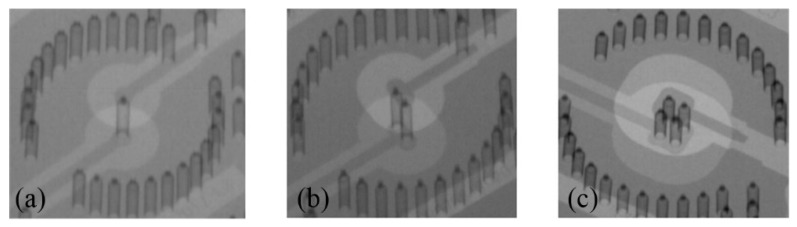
Test structure X-ray detection map: (a) single TSV; (b) dual redundant TSV; (c) quad redundant TSV.
